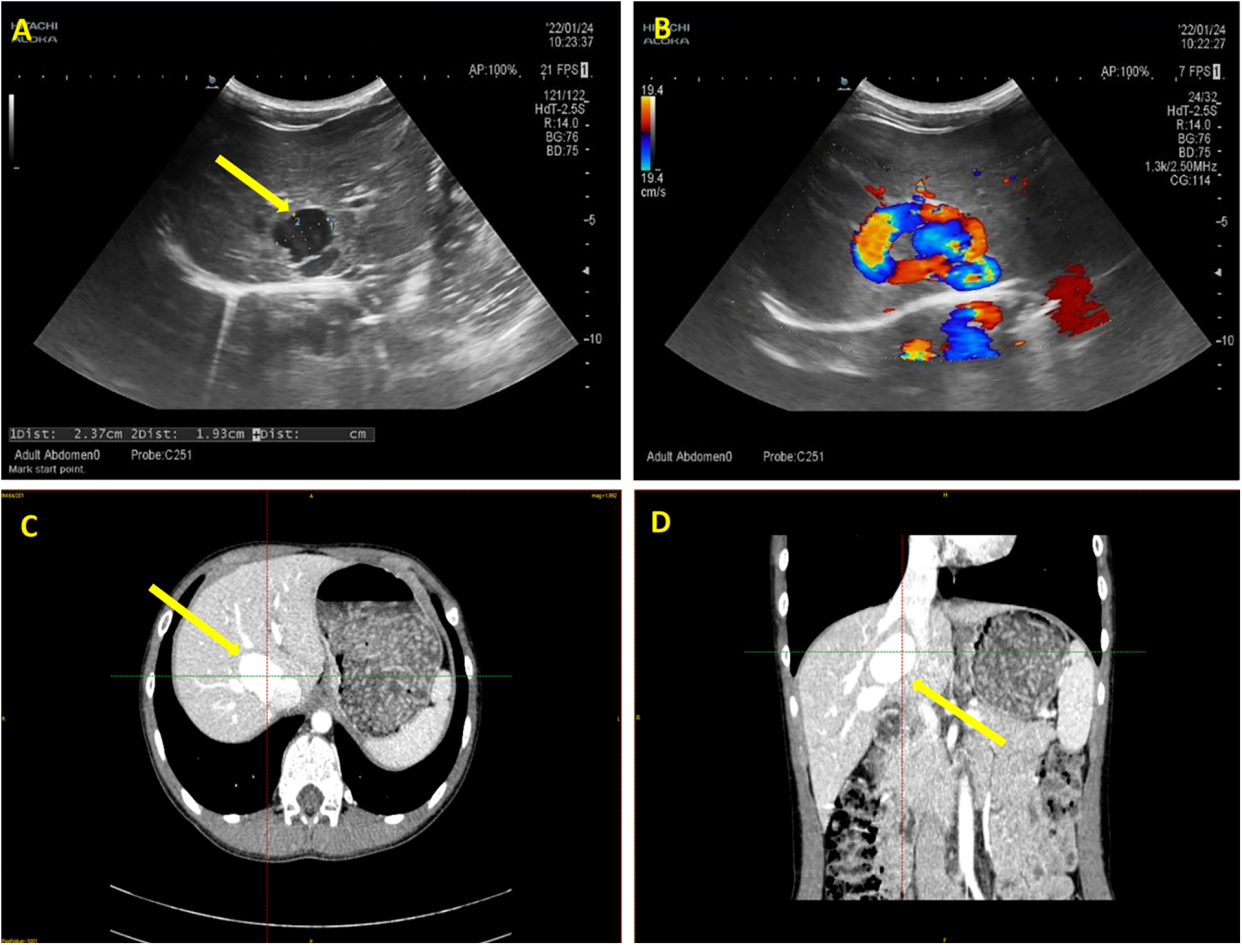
Ultrasonography and enhanced computed tomography (CT) imaging of the patient abdomen. (A) The arrow highlights the tumor-like dilatation of the portal vein, demarcated by distinct boundaries. (B) Color Doppler ultrasound imaging verifies the directional flow from the portal vein to the inferior vena cava, with the flow velocity of 68.0 cm/s. (C) Transverse section and (D) Coronal section of enhanced CT images. The arrows indicate the tortuously dilated portal vein.
